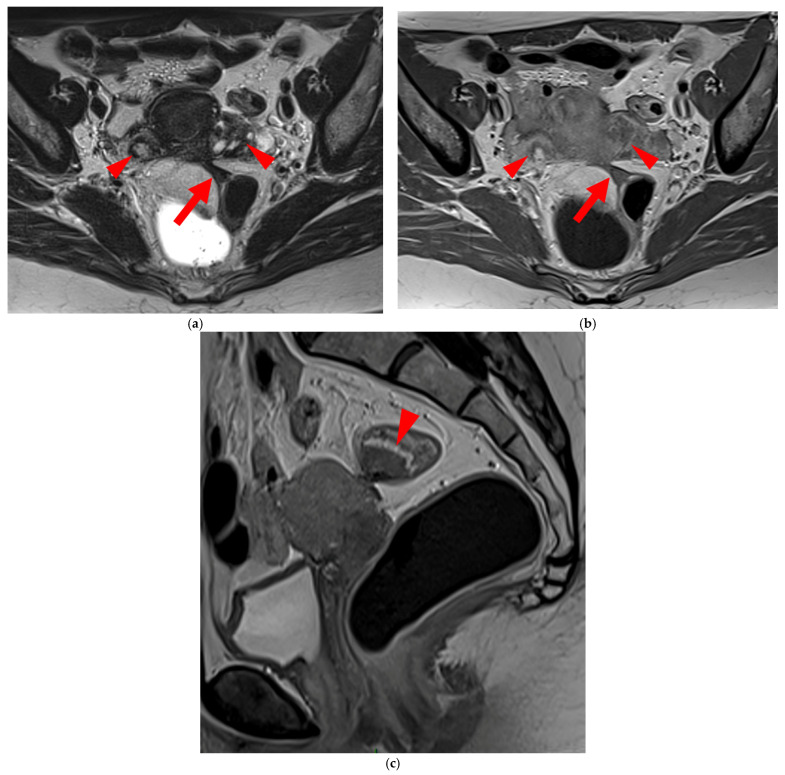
Pelvic MRI scan of a patient with a “kissing ovaries” sign and rectal involvement (i.e., HTD type 6 USLs). (a,b) Axial T2WI (a) and contrast-enhanced T1WI (b) show both ovaries in close proximity (“kissing ovaries” sign) (*arrowheads*) and rectosigmoid wall infiltration (*arrows*). (c) Sagittal contrast-enhanced T1WI shows rectosigmoid wall infiltration, appearing as a “medallion-shaped” lesion outlined by markedly enhancing mucosa (*arrowhead*).
